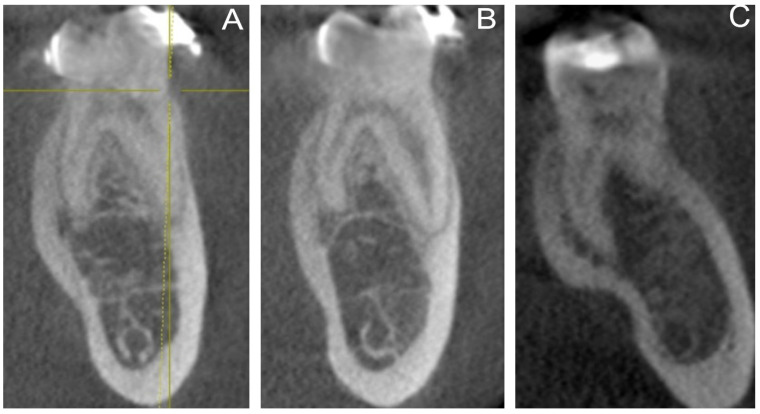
Coronal section of a CBCT image indicating the three variants of radix entomolaris according to Song et al.: (A) type 1, (B) type 2 and (C) type 3.
